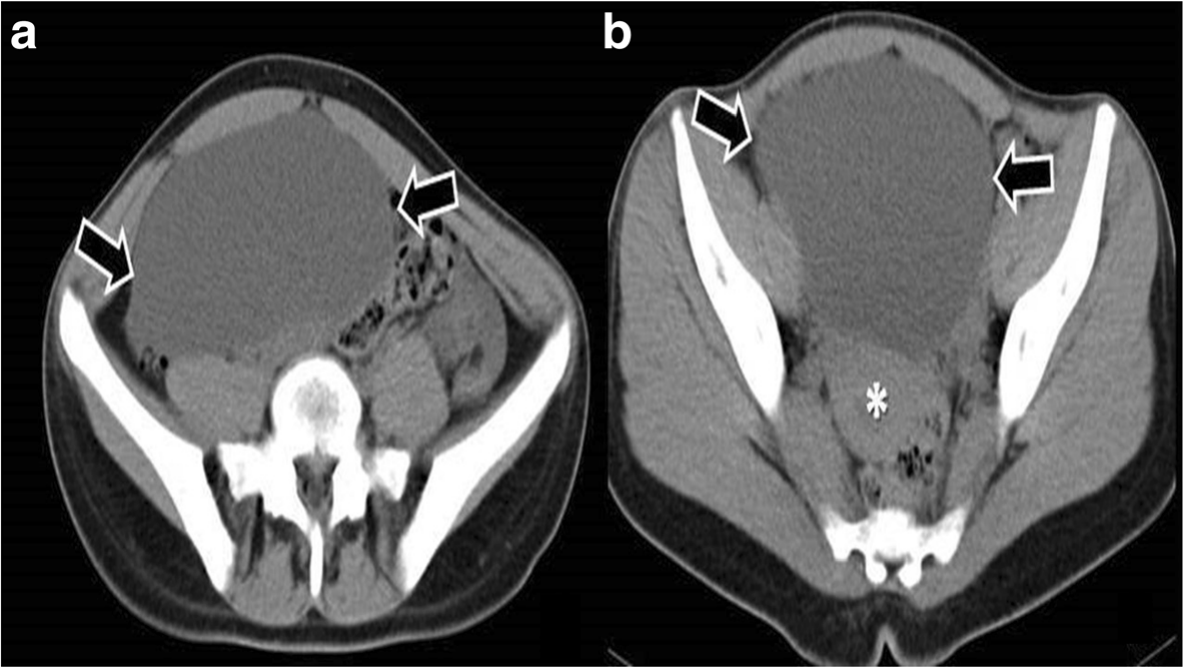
a and b: axial, unenhanced CT images of the pelvis demonstrate a voluminous mass [arrows] which displace the adjacent viscera [small intestine and uterus (star)]
